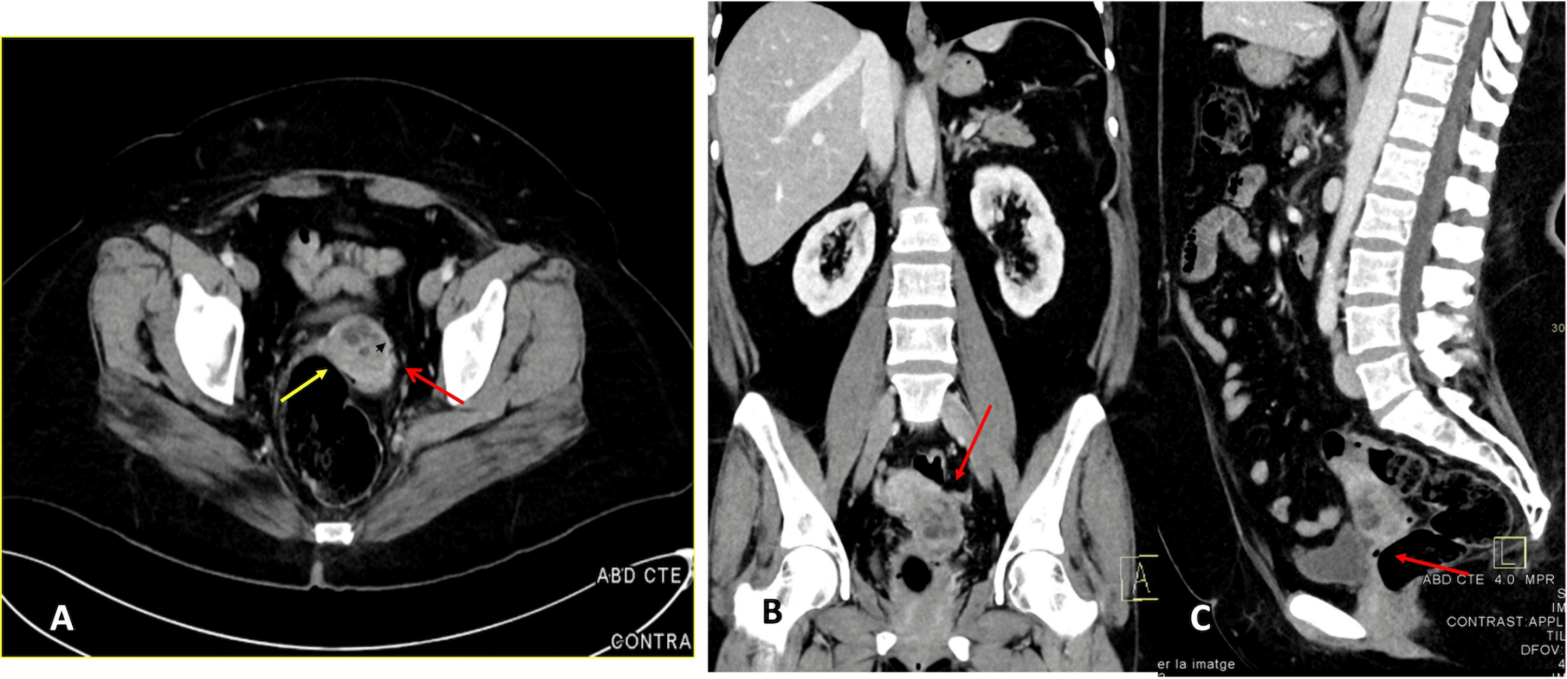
Computed tomography (CT) A. Axial CT+C portal venous phase CT features a soft tissue mass with heterogeneous enhancement (measuring 44x40 mm) centered on the uterine cervix, located between the yellow and red arrow. No abnormal pelvic or para-aortic lymph nodes. B. Coronal CT + portal venous phase. It appears to invade the uterine cavity, indicated by the red arrow. C. Coronal CT + portal venous phase. There is no apparent extension to the anterior rectal wall or the posterior wall of the bladder, as indicated by the red arrow.
